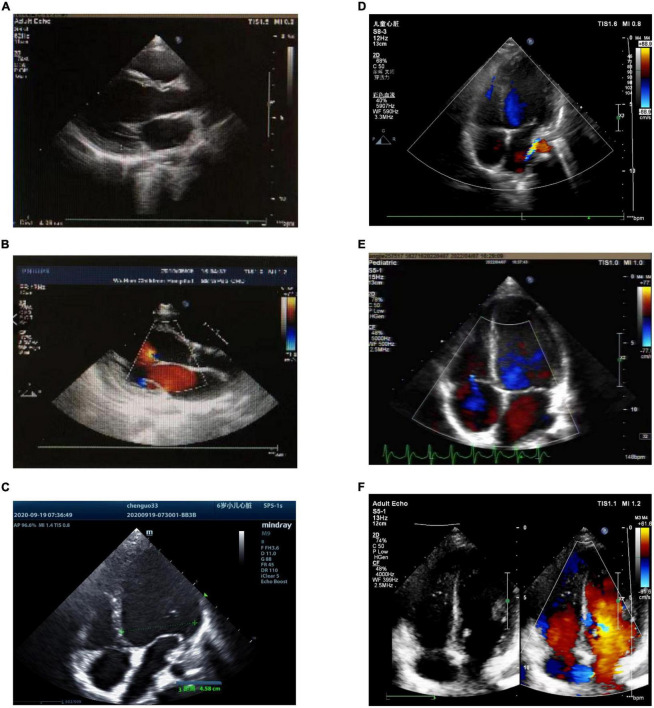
Preoperative and postoperative echocardiography of atrial tachycardia (AT) from the atrial appendage. (A) Case 1: preoperative echocardiography. Left ventricular end-diastolic diameter (LVEDD) 40 mm, left ventricular ejection fraction (LVEF) = 42%. (B) Case 1: echocardiography 1°month after radiofrequency catheter ablation (RFCA). LVEDD 37 mm, LVEF = 53%. (C) Case 2: preoperative echocardiography. LVEDD 45.8 mm, LVEF = 29%. (D) Case 2: echocardiography 1°month after LAA resection. LVEDD 37 mm, LVEF = 46%. (E) Case 3: the left picture shows preoperative echocardiography. LVEDD 45 mm, LVEF = 45%. (F) Case 3: echocardiography 1°month after RAA resection. LVEDD 42 mm, LVEF = 56%. LVEDD, left ventricular end-diastolic diameter; LVEF, left ventricular ejection fraction; RFCA, radiofrequency catheter ablation; LAA, left atrial appendage; RAA, right atrial appendage.
